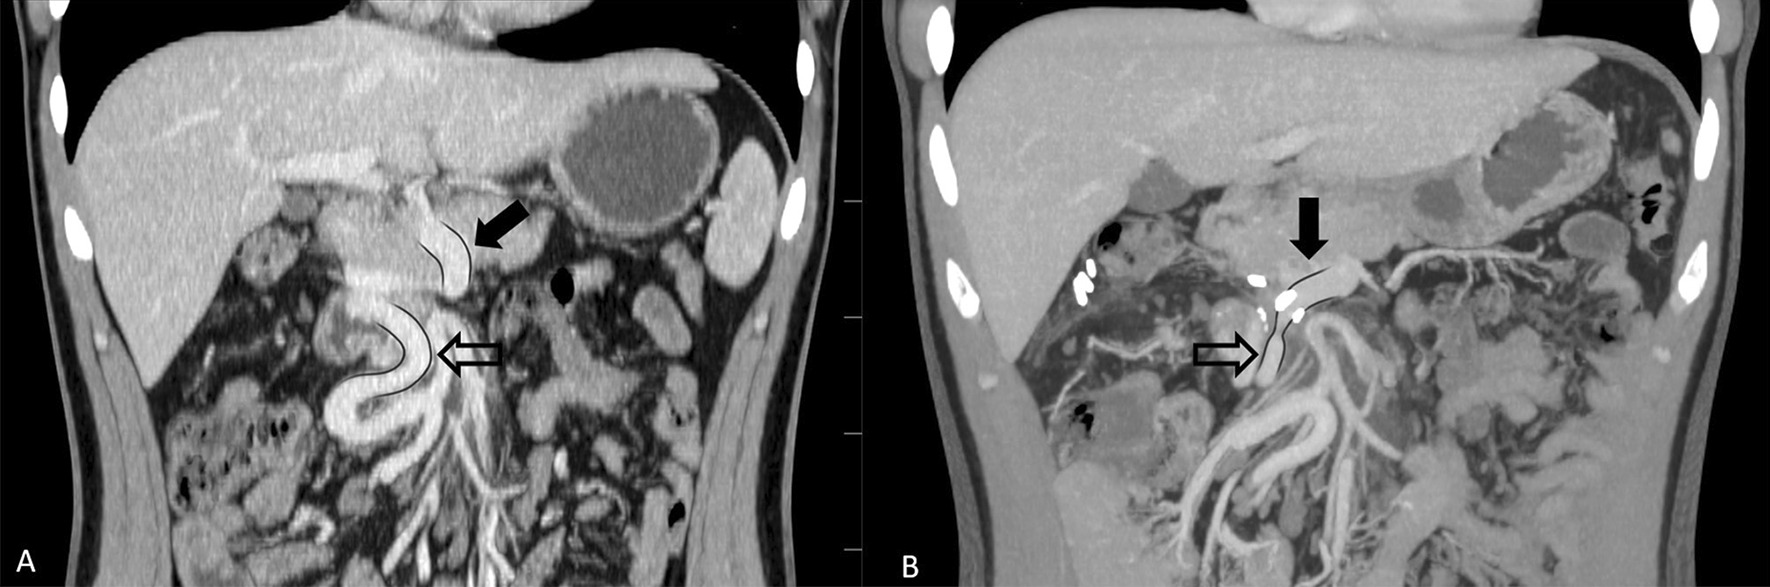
Abdominal CT—coronal sections. A Preoperative CT: the full black arrow points to the proximal section of the SMV, the contoured black arrow points to a large collateral arising from the distal section of the SMV. B Postoperative CT shows patent anastomosis between collateral branch from the distal section of the SMV and the anterior inferior pancreaticoduodenal vein
